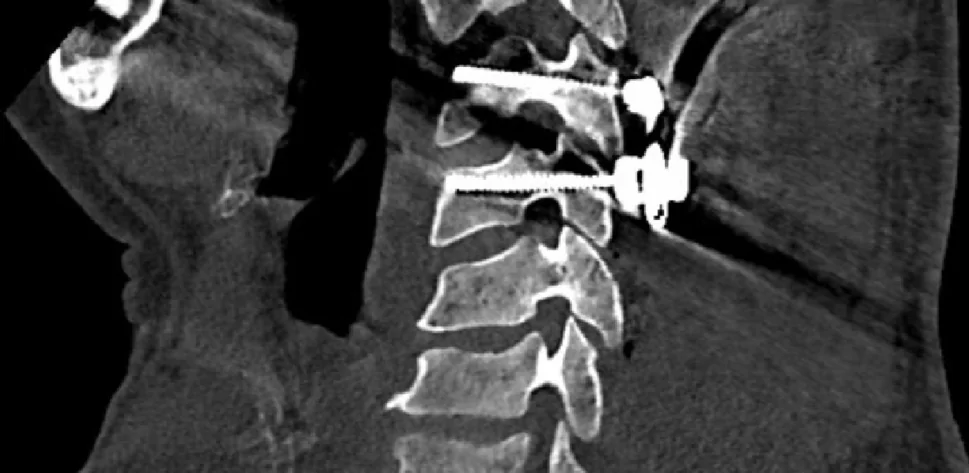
Sagittal CT pedicle screw C4-rt. (same patient as in figures 3–7 and 9). *C*,* cervical spine*
